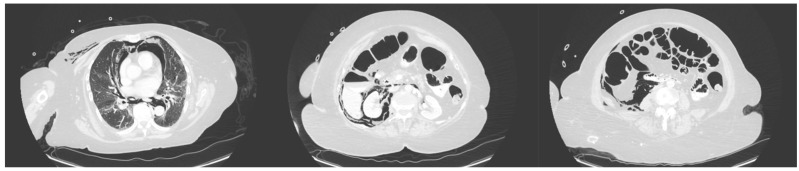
The presence of pneumoretroperitoneum, pneumoperitoneum and pneumomediastinum on CT imaging in a patient with an ERCP-associated Stapfer II perforation.
